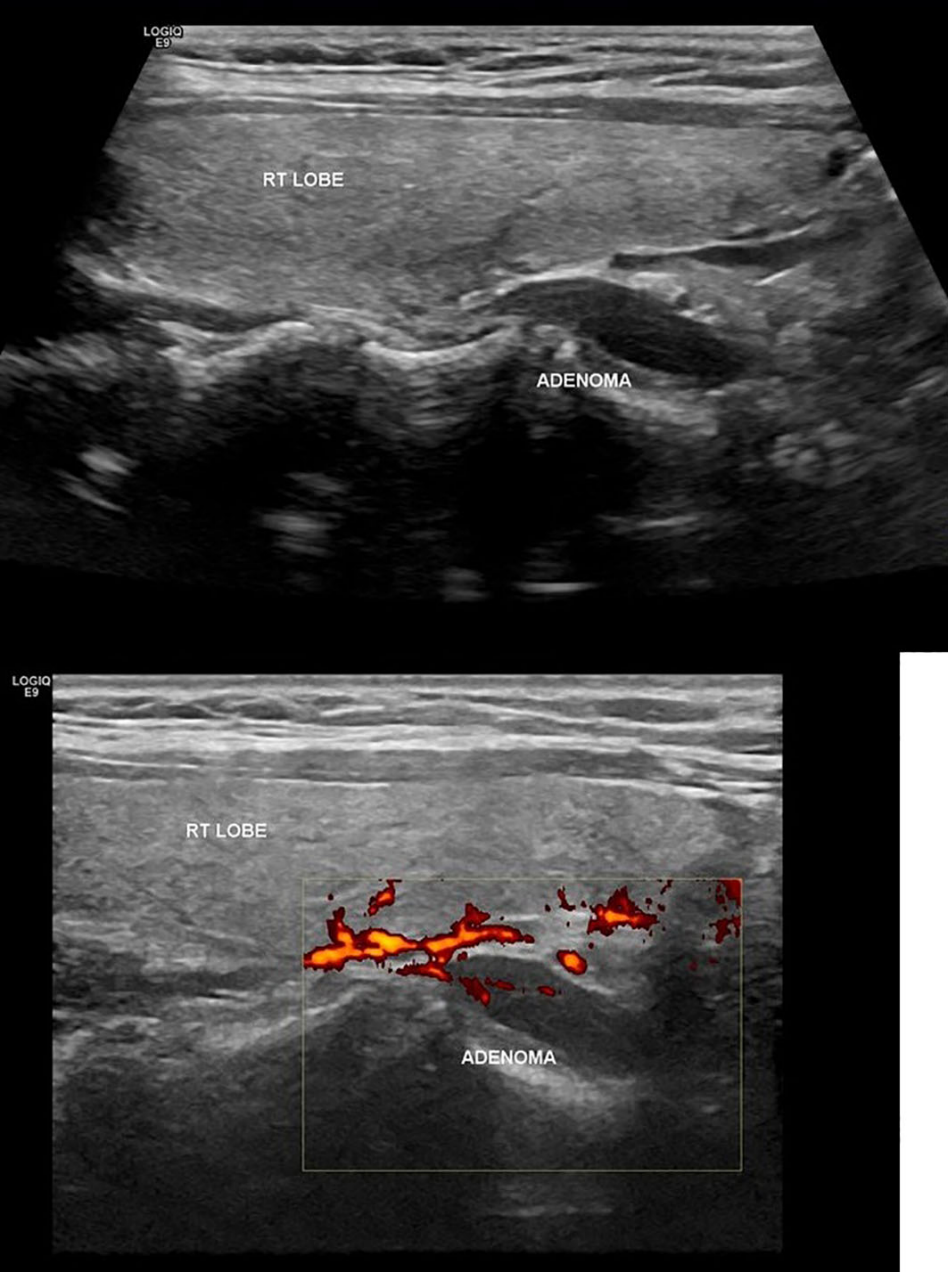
Ultrasonographic findings of parathyroid adenoma.
